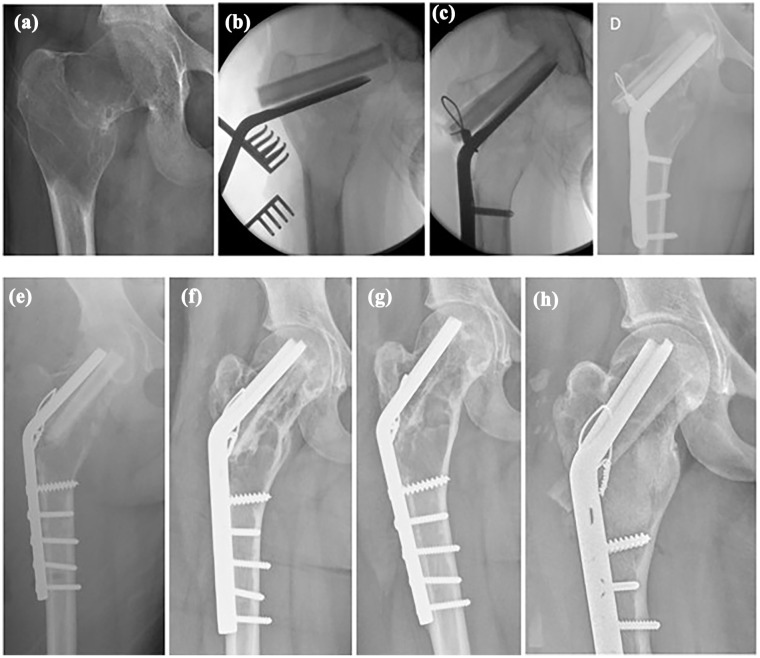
A 15-year-old girl presenting with pain, (a) on radiographs cystic degenerated monostotic fibrous dysplasia in the right proximal femur with neck–shaft angle of 113°. (b) Intraoperative imaging, 120° angled blade plate with two fibular strut grafts places cranially. (c) Pulling screws brought the distal part of the plate toward the femur shaft, while lifting the femoral neck into a normal neck–shaft angle, without osteotomy. Cerclage was conducted for added stability. (d) One day postoperative, there was protrusion of the angled blade plate into the intra-articular space due to soft bone. (e) Revision, 2 days post-original operation with shorter 130° blade plate and more distal screws. (f) After 8 months, the consolidation of the cyst with resorption of the strut graft. (g) One year post-operation, the strut graft is fully resorbed, but there is thickening of the cortices. (h) Last follow-up, patient returned after 6 years and 3 months with pain and abnormal gait, for which new cortical strut grafts were placed along with Vitoss synthetic cancellous bone filling. After this, there were no more symptoms.
